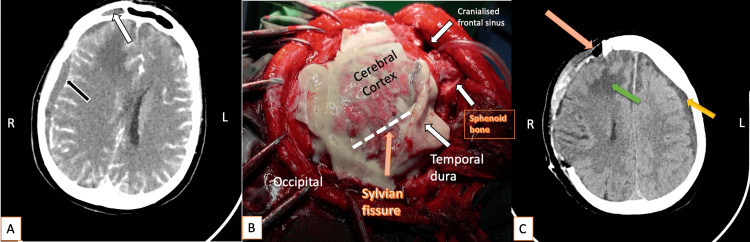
(A) Preoperative contrast-enhanced CT demonstrates a right-sided subdural collection (black arrow) and a collection within the right frontal sinus (white arrow). (B) Intraoperative findings reveal purulent material following cruciate dural opening, with hyperemic cortical surface and encephalitic changes. The Sylvian fissure is indicated by an orange arrow, and the inferiorly displaced temporal dura by a white arrow. (C) Early postoperative CT (third postoperative day) shows frontal sinus cranialization (beige arrow), CT signs of early frontal cerebritis (green arrow), marked cerebral edema, and a contralateral subdural collection measuring approximately 8 mm (yellow arrow), with restored midline alignment. CT = computed tomography; L = left; R = right
